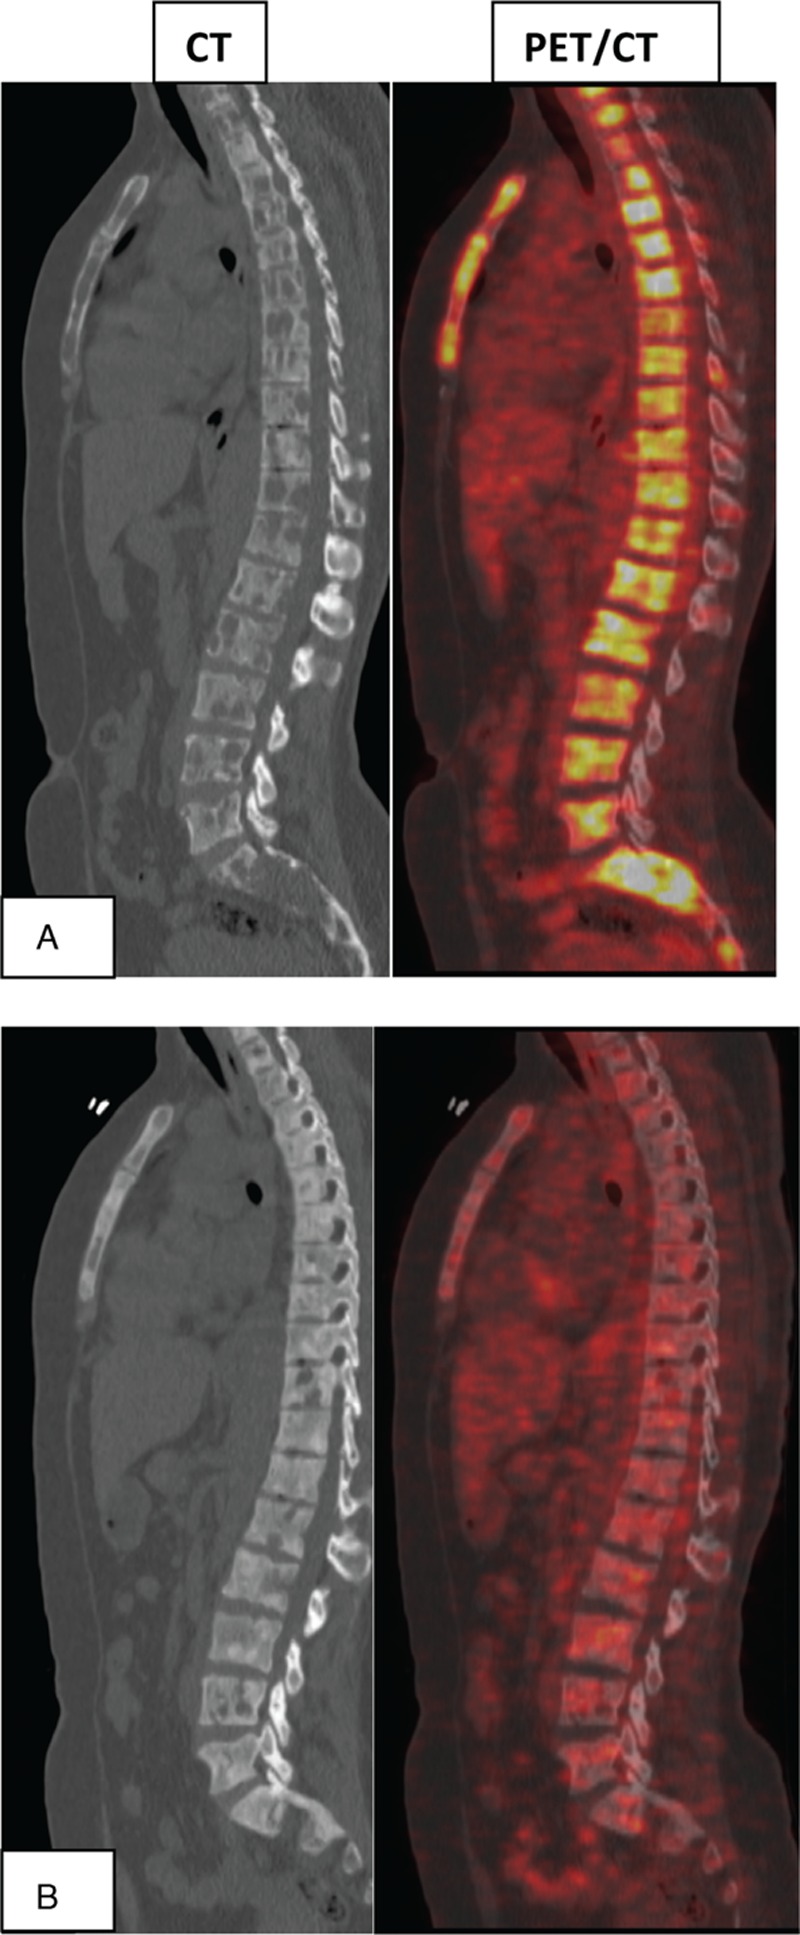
Multiple 18F-FDG-positive osteolytic metastatic lesions on baseline PET/CT (A) followed by complete response to treatment evidenced by total disappearance of 18F-FDG focal activity on follow-up PET/CT with ossification of osteolytic lesions that became osteoblastic lesions on CT images (B). 18F-FDG = fluorine-18 fluoro-2-deoxy-D-glucose, PET/CT = positron emission tomography/computed tomography.
